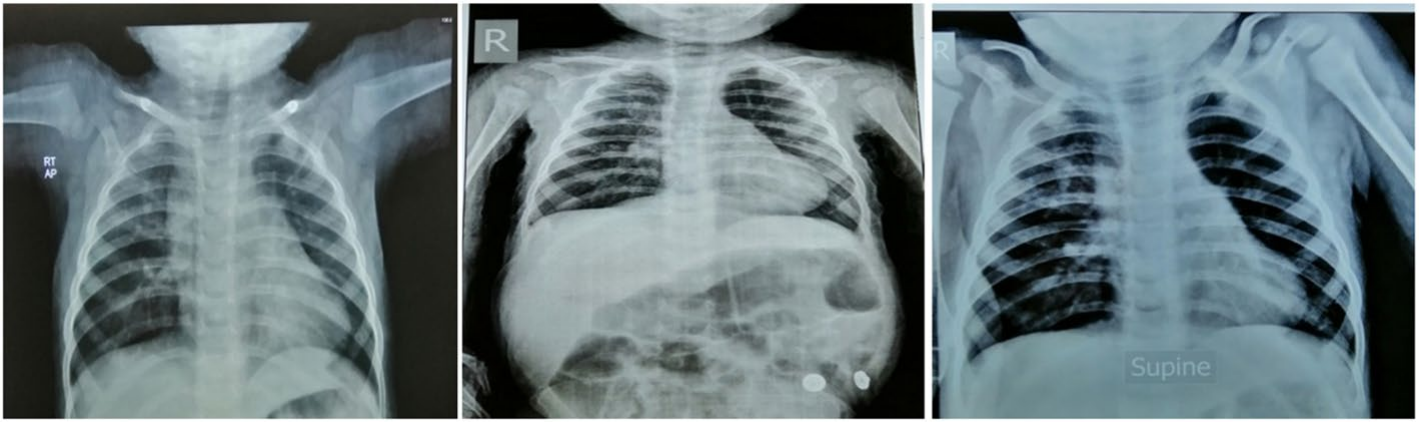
Plain chest radiographic examination showing patchy infiltrates in the lungs
